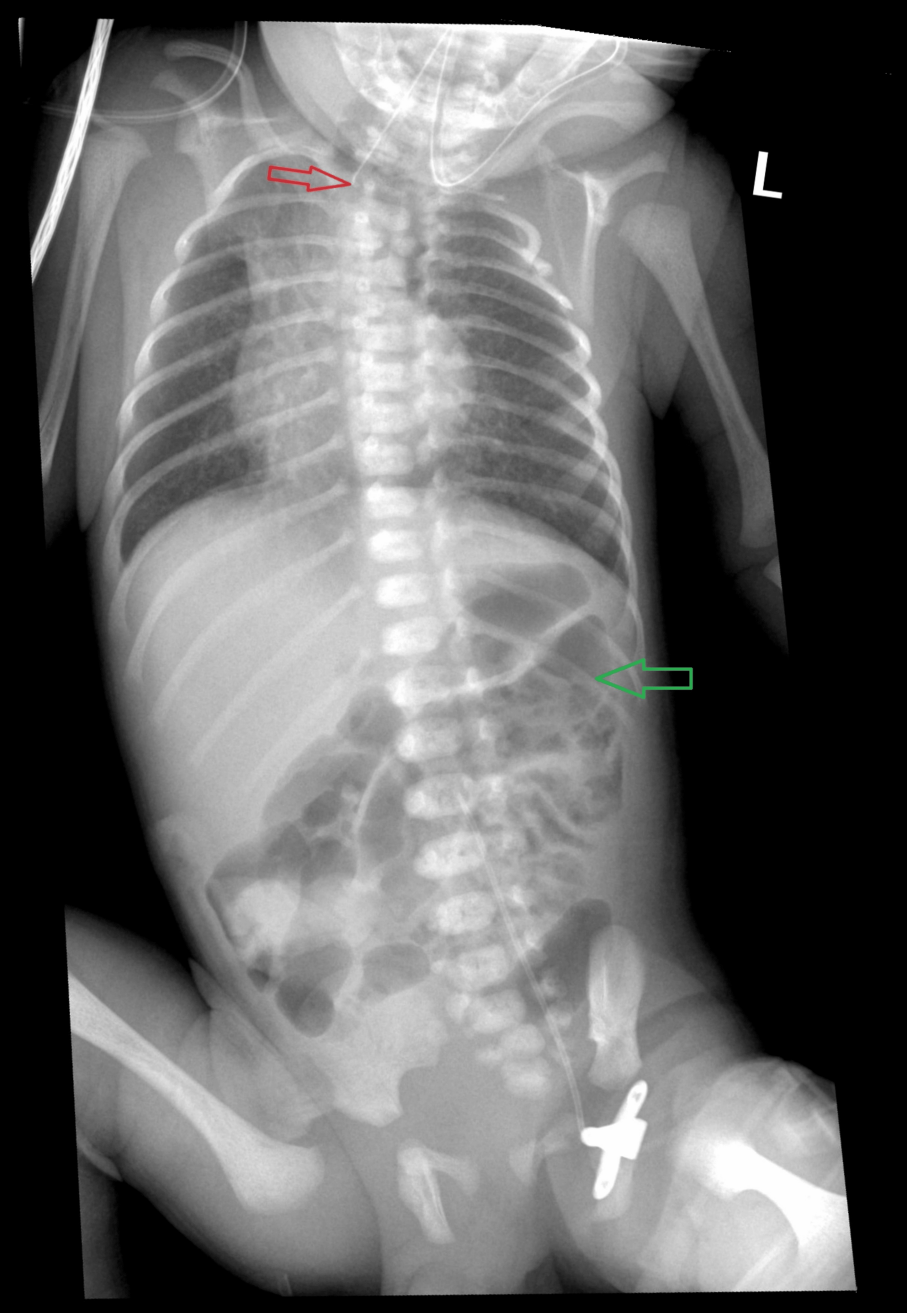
The after-birth chest and abdomen X-ray The red arrow points to the end of the gastric probe at cervical 7 /thoracic 1( C7/Th1 ) level. The green arrow points to the air in intestinal loops.
